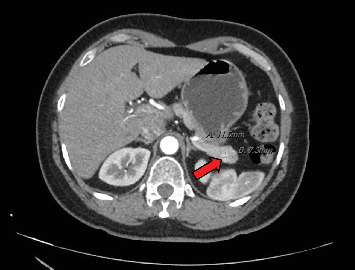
Case 3 radiographic findings. CT abdomen and pelvis with contrast showed a 1.2-cm arterial enhancing mass in the pancreatic tail (arrow). There was no pancreatic ductal dilatation, and the remainder of the pancreas showed normal enhancement.
